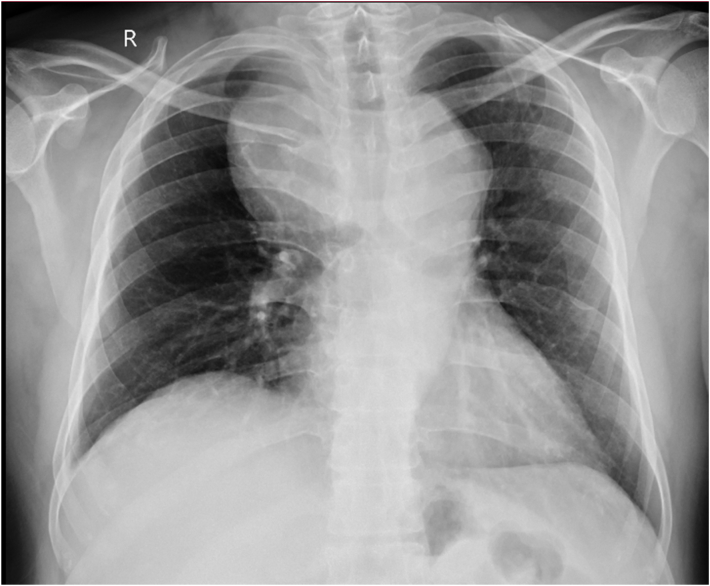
Chest X-ray of the patient showing a wide mediastinum.
